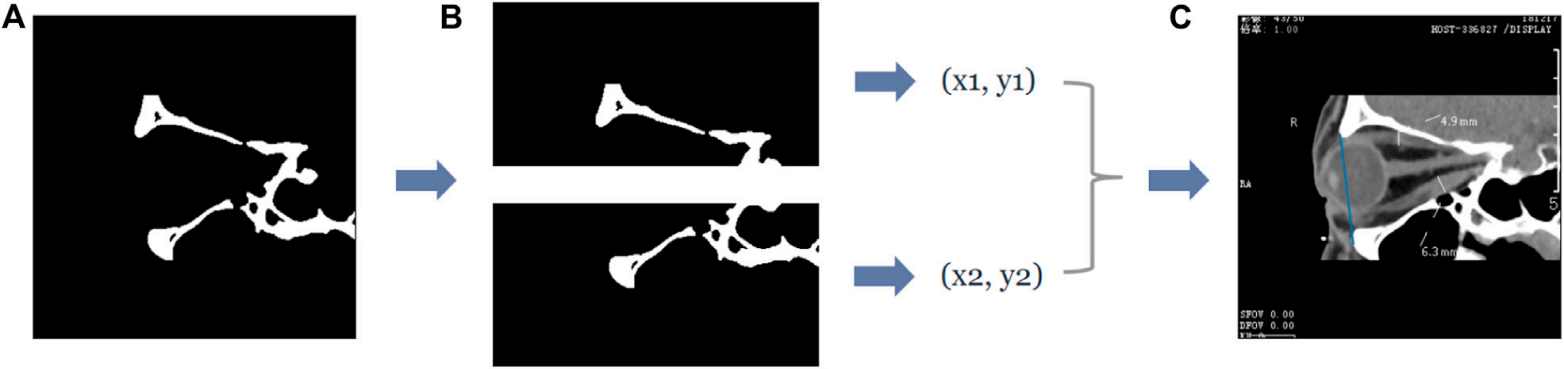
The process of obtaining the coordinates of the most protruding points of the upper and lower orbital rim in the sagittal plane of the CT image. (A) The second row of Figure 4B, which is the binary image containing the upper and lower orbital rim regions, and extracts the submaps of the upper and lower halves, respectively, to obtain the two submaps as shown in (B). Subsequently, the coordinates of the most protruding points of the superior and inferior orbital rims can be determined by traversing the two subgraphs separately, and then the straight line passing through the two most protruding points can be obtained—the straight line is shown in blue in (C).
